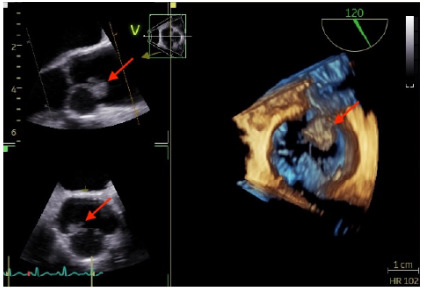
Transesophageal echocardiography showing a 1.5 × 1.0 centimeters, highly mobile lesion attached to the left coronary cusp of the aortic valve (arrows). The mass has a very small neck and intermittently obstructed the left main coronary ostium.
